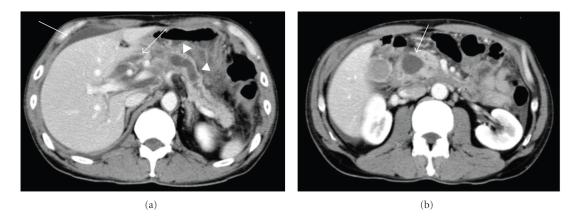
Enhanced CT image. (a) The dilated main pancreatic duct (PD) (arrowhead) and fluid collection (arrow) surrounding the pancreas and the liver were demonstrated. (b) A pseudocyst (arrow) at the pancreatic head was revealed.
